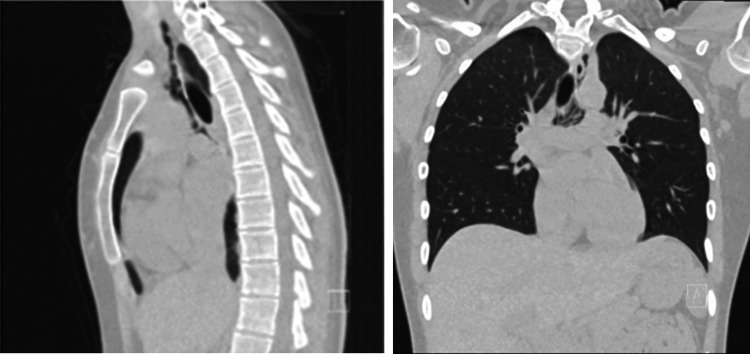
CT images confirm the presence of pneumomediastinum in sagittal (left panel) and coronal (right panel) views. No associated complications or pleural effusion are seen.
